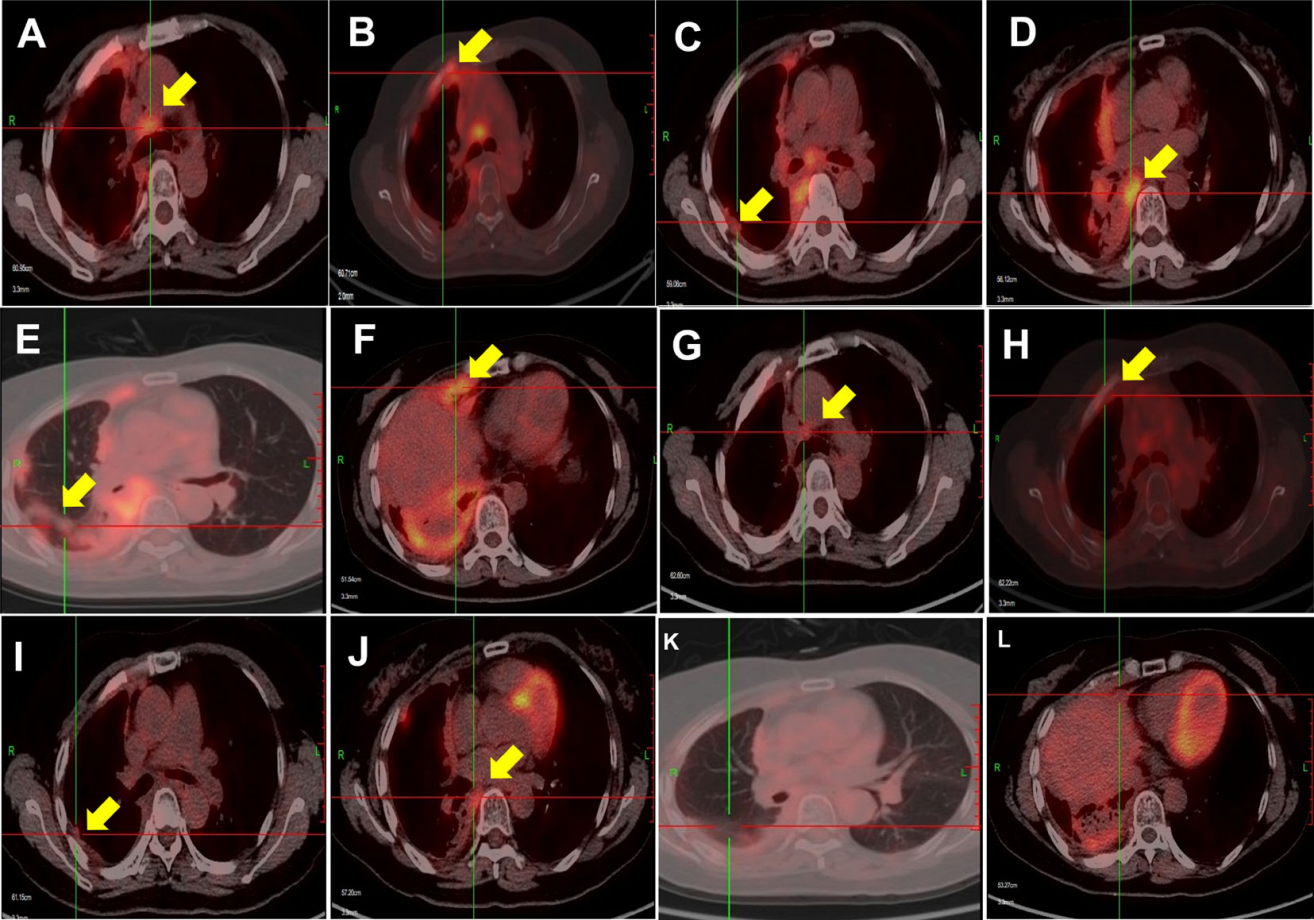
PET/CT scans showing disease response to lenvatinib treatment. The lesions in the right lung and right pleura were substantially smaller, and the metabolism of the lung, pleural, and bone metastases was decreased in March 2021 (G–L) compared with that in September 2020 (A–F).
